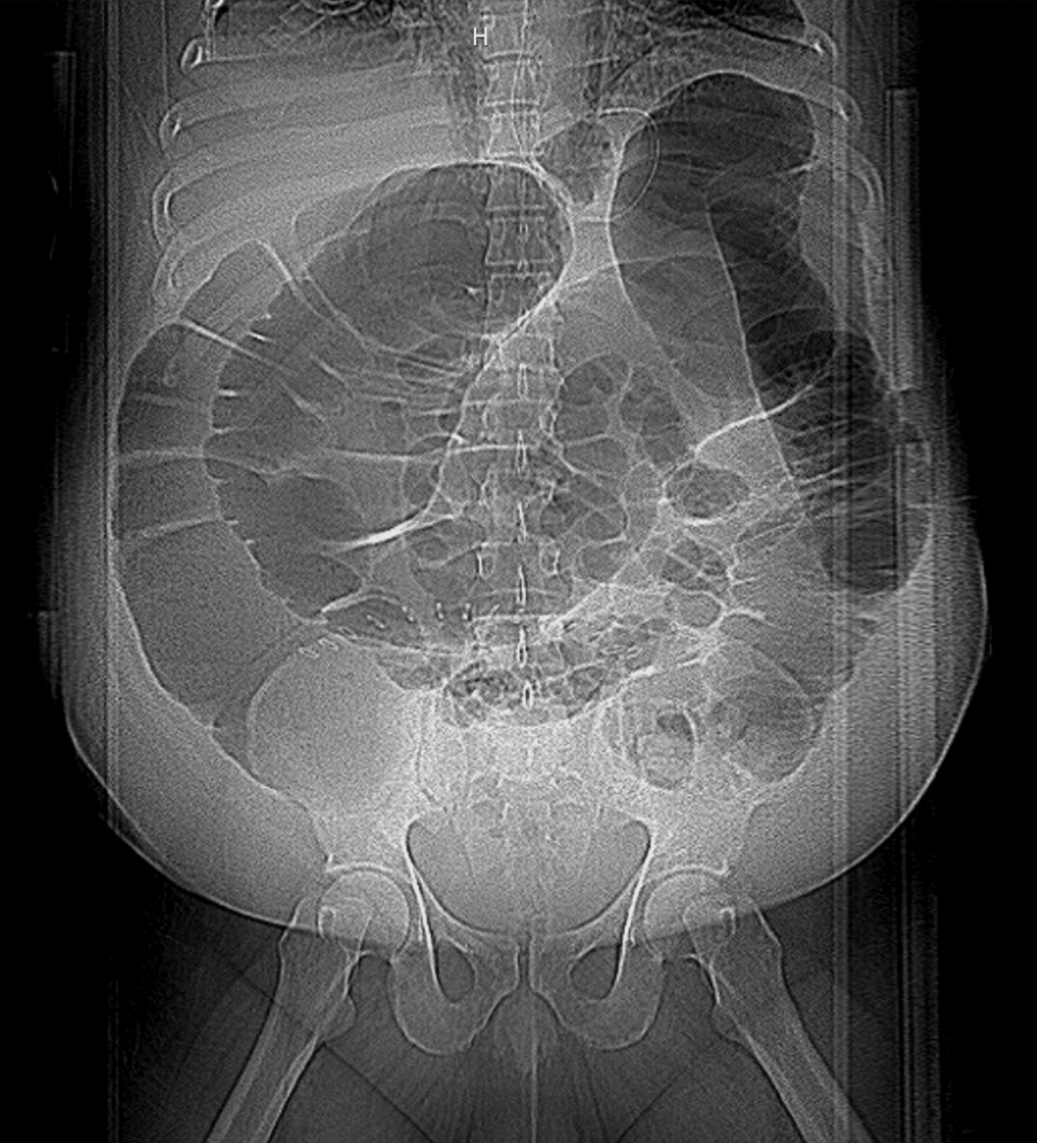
Abdominal X-ray showing dilated large intestine
